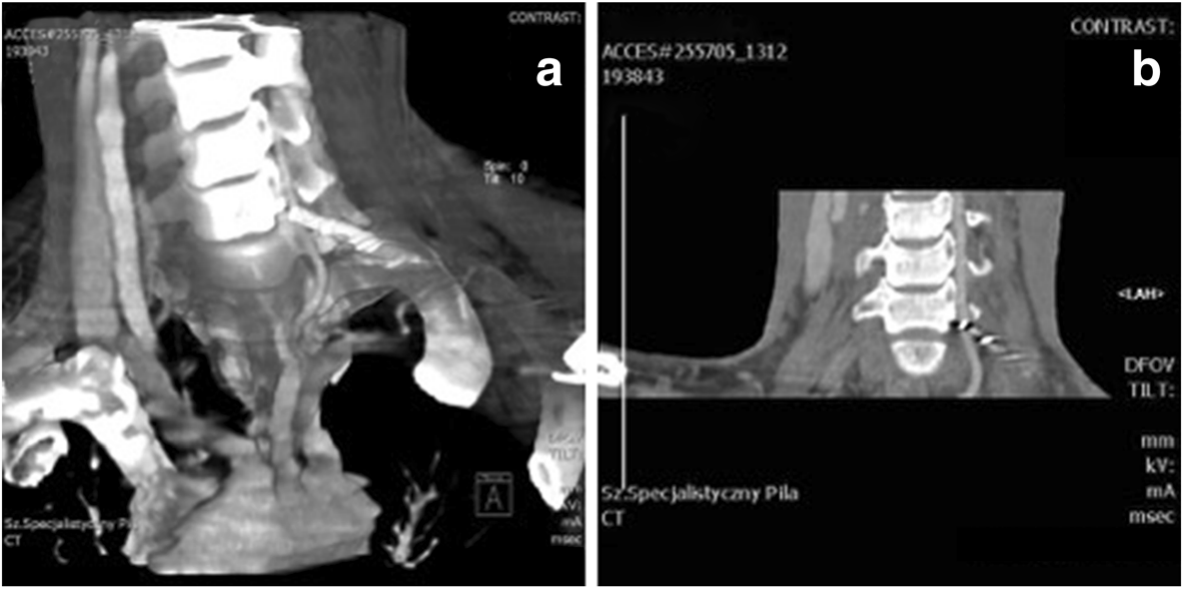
a Angio-computed tomography of cervical spine; Kirschner wire penetration of C6 vertebra. b Angio-computed tomography of cervical spine; direct contact of Kirschner wire with vertebral artery without breaching the lumen
